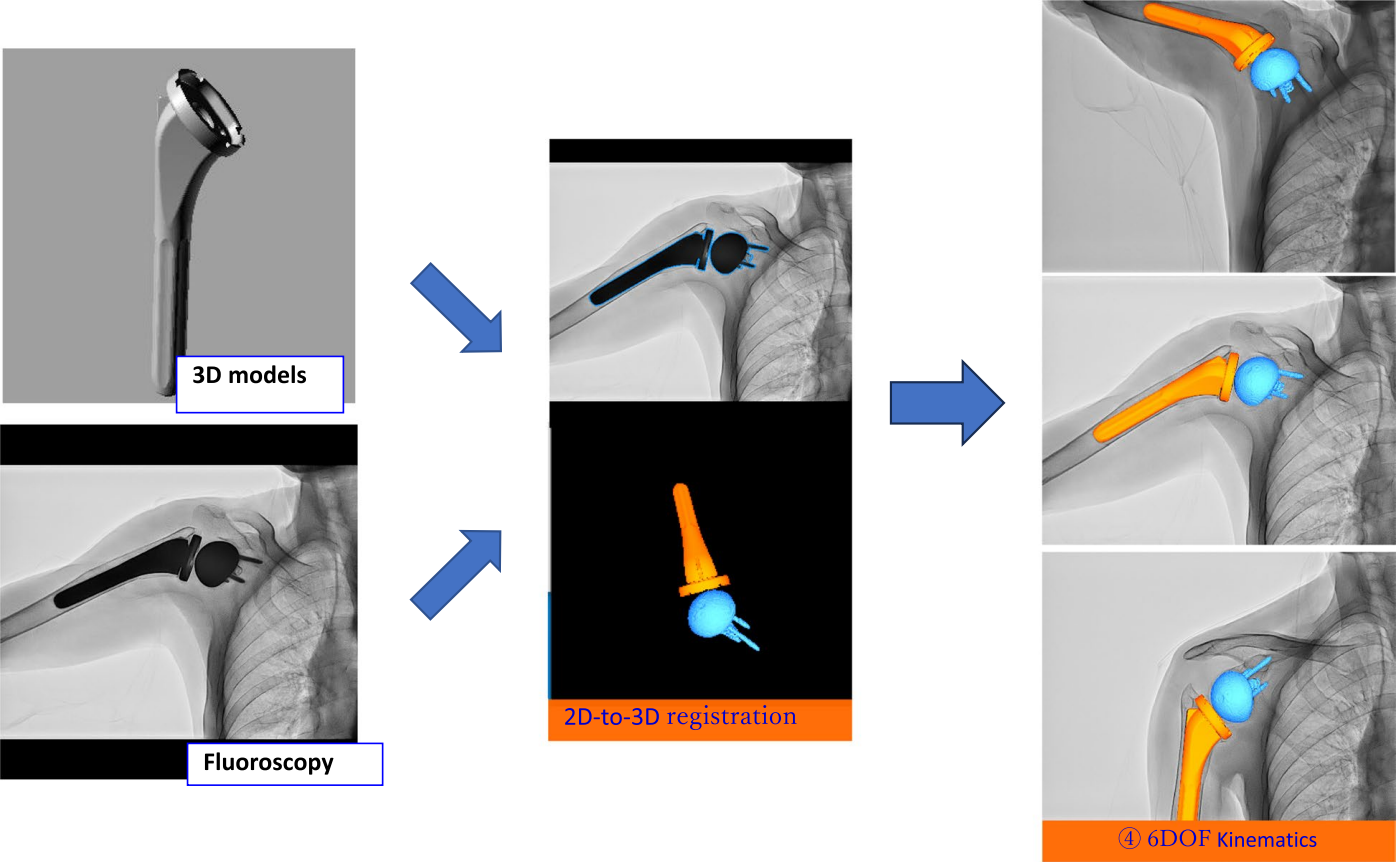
The 2-dimensional-3-dimensional model image registration in RTSA. Three-dimensional artificial models were fitted to the contour of the fluoroscopic X-ray image
